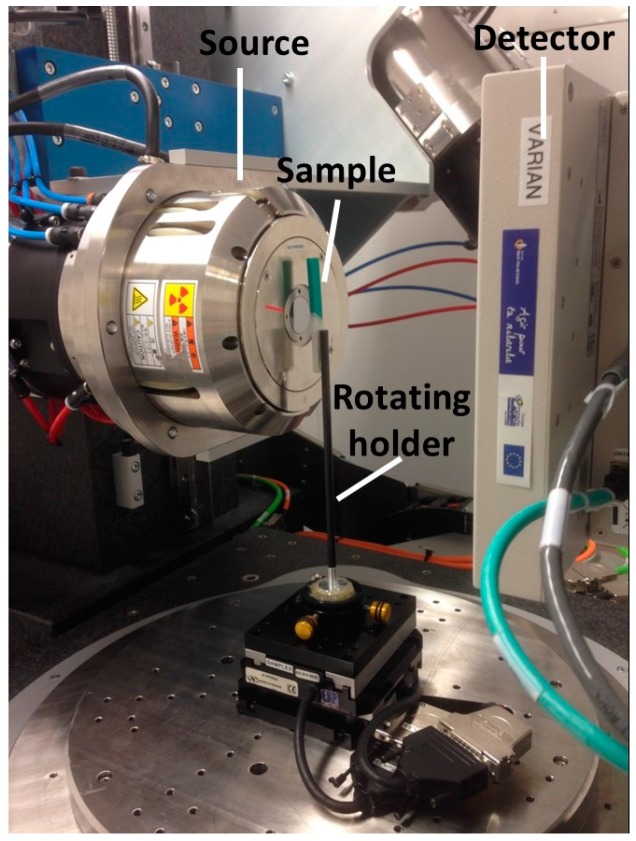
X-ray micro-tomography setup to acquire three-dimensional (3D) microstructural images of multi-material printed slabs.
